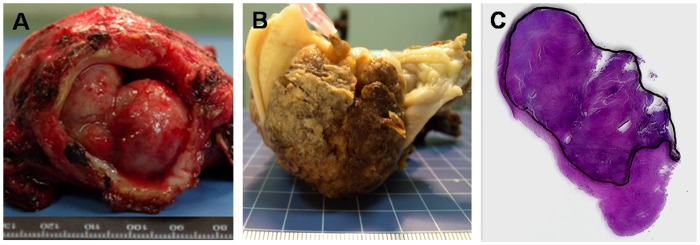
Determination of the optimal standardized uptake value (SUV) cut-off value. Data from case 6 is shown as a representative example. The serial gross tumor volumes on positron emission tomography (GTV_PET_) measured on the basis of each cut-off value (A, SUV = 1.5, 2.5, 3.5, 4.5 and 5.5; B, % SUV = 10, 20, 30, 40 and 50) are plotted according to the threshold relative to the absolute SUV (C) or percentages of maximal SUV (D). The optimal cut-off value is determined by the linear approximation with the best agreement between the GTVPET and pathologically determined GTV (GTV_path_).
